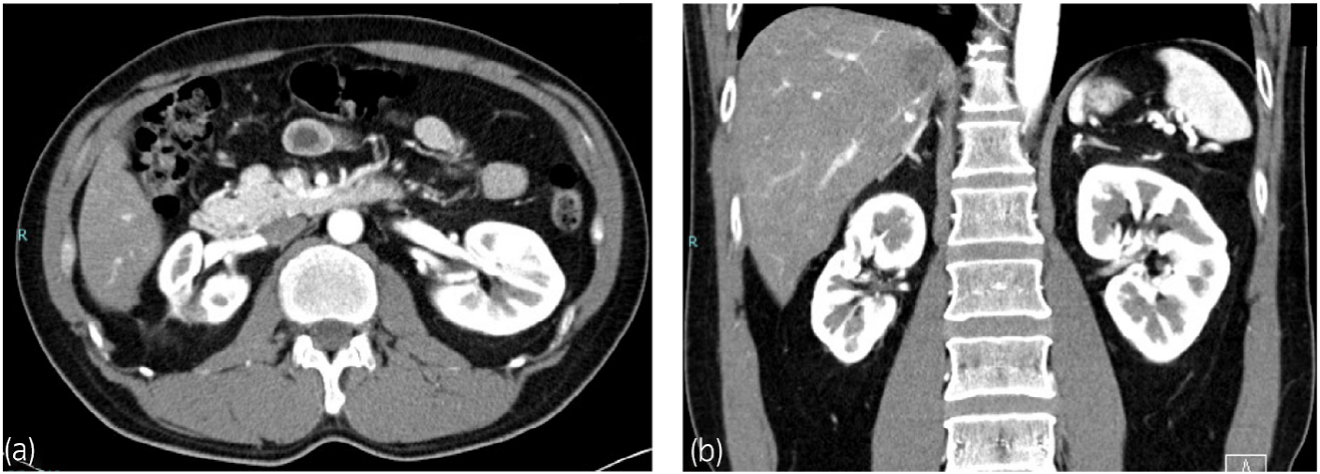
A computed tomography image obtained at two and a half postoperative years. There are no findings suggestive of a urinary fistula or RCC. (a) Coronal section, cortex phase, (b) Axial section, cortex phase.
